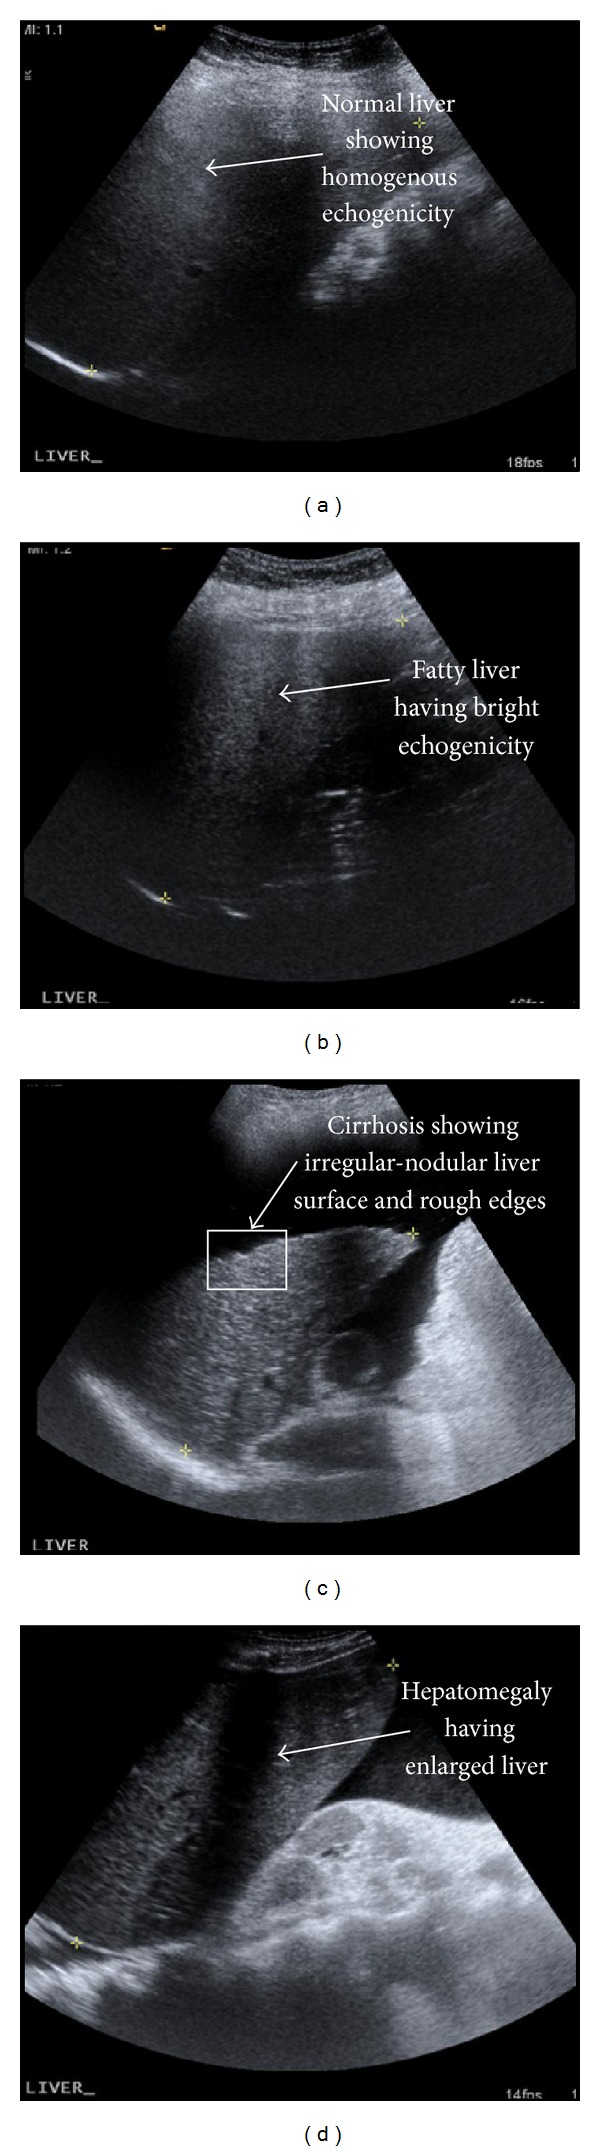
Ultrasound image of (a) normal liver, (b) fatty liver, (c) cirrhosis, and (d) hepatomegaly.
